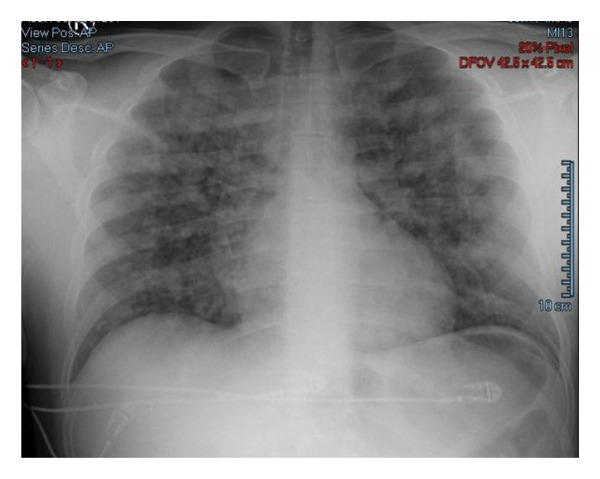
Chest radiograph showing diffuse bilateral pulmonary infiltrates compatible with acute pulmonary edema. Arrows show increased pulmonary vascular congestion.
